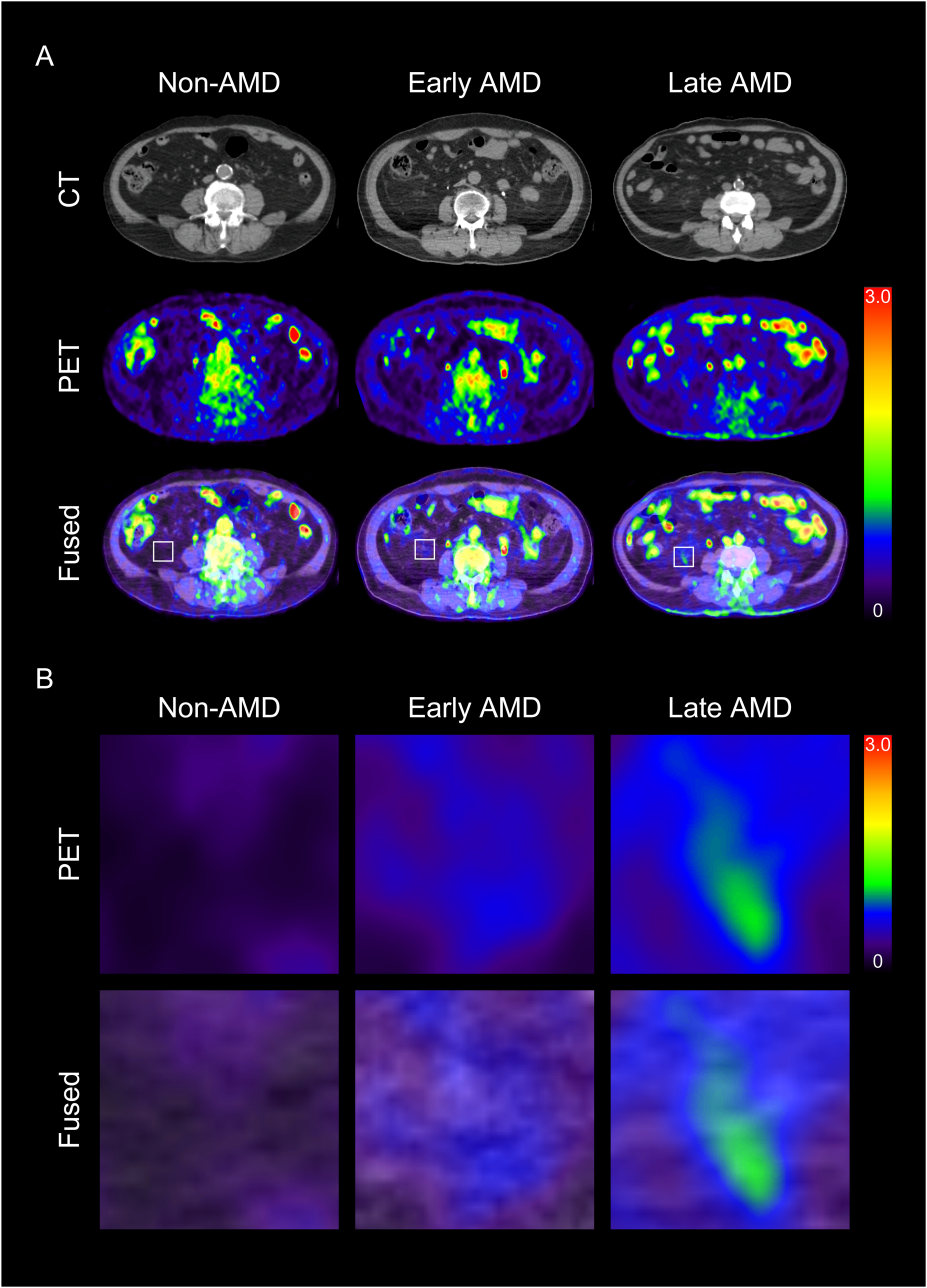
Representative images of visceral adipose tissue (VAT) metabolic activity according to the severity of age-related macular degeneration (AMD) (A) and their corresponding magnified images (B). CT, computed tomography; PET, positron emission tomography.
